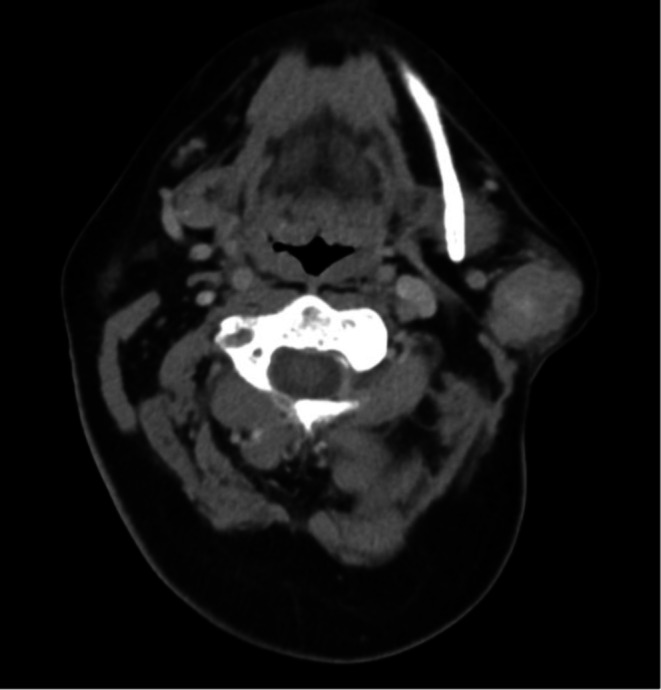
CT soft tissue head and neck displaying parotid tail lesion.
